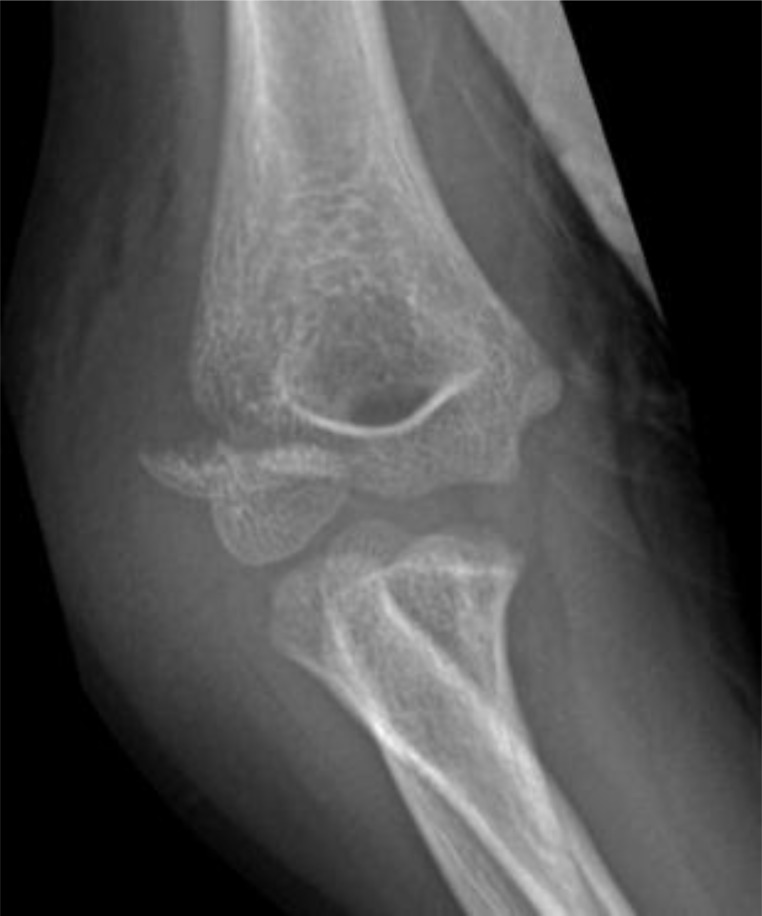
AP radiograph of a Type 3 Jakob Classification lateral condyle fracture.
